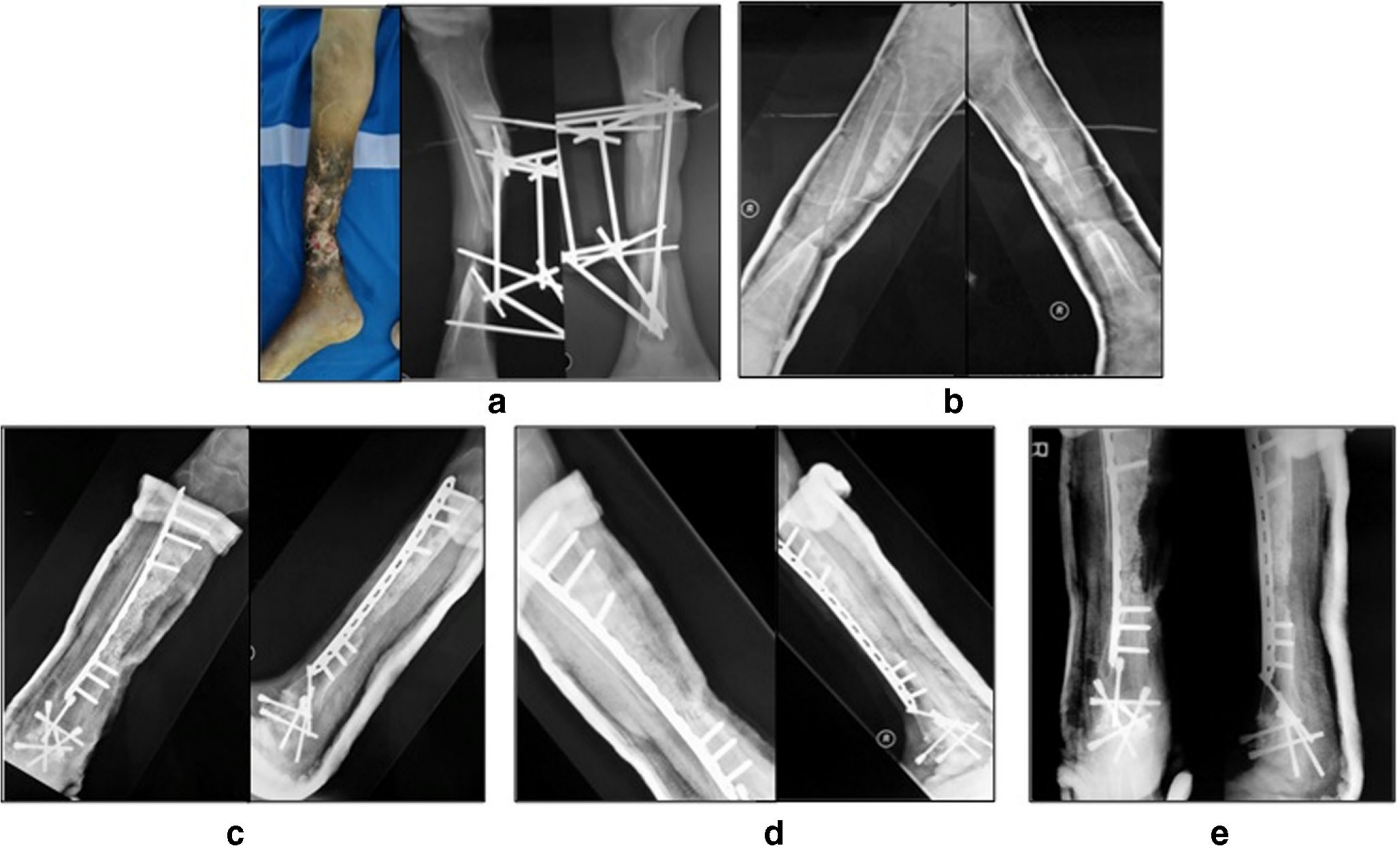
A 28-year-old male (case 4) with 7-year history of 8-cm bone defect of the right tibia. a Initial x-ray and clinical picture after external fixation removal. b Pre-operative radiography. c Post-operative, d Five-month radiography. e Twelve-month radiography
